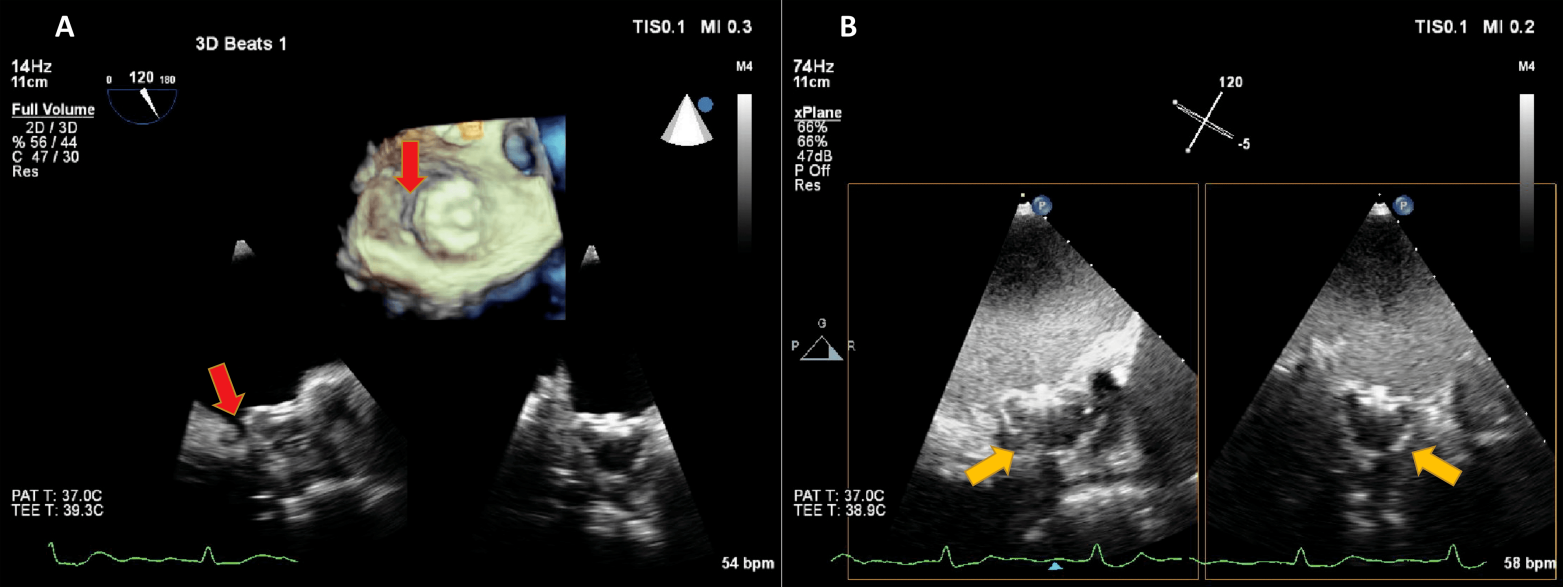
Transoesophageal echocardiography in a patient with a Watchman
